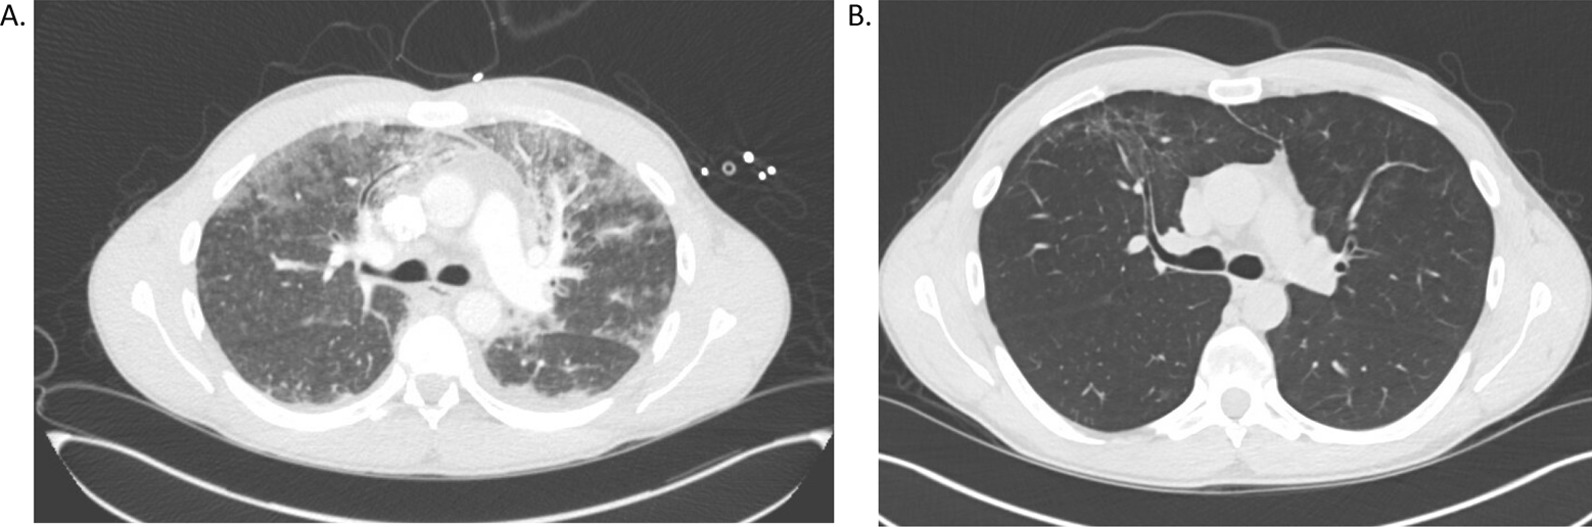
Representative CT images from A CTA chest obtained during initial hospitalization, and B follow up high-resolution CT scan (HRCT) obtained 6 weeks after hospital discharge
